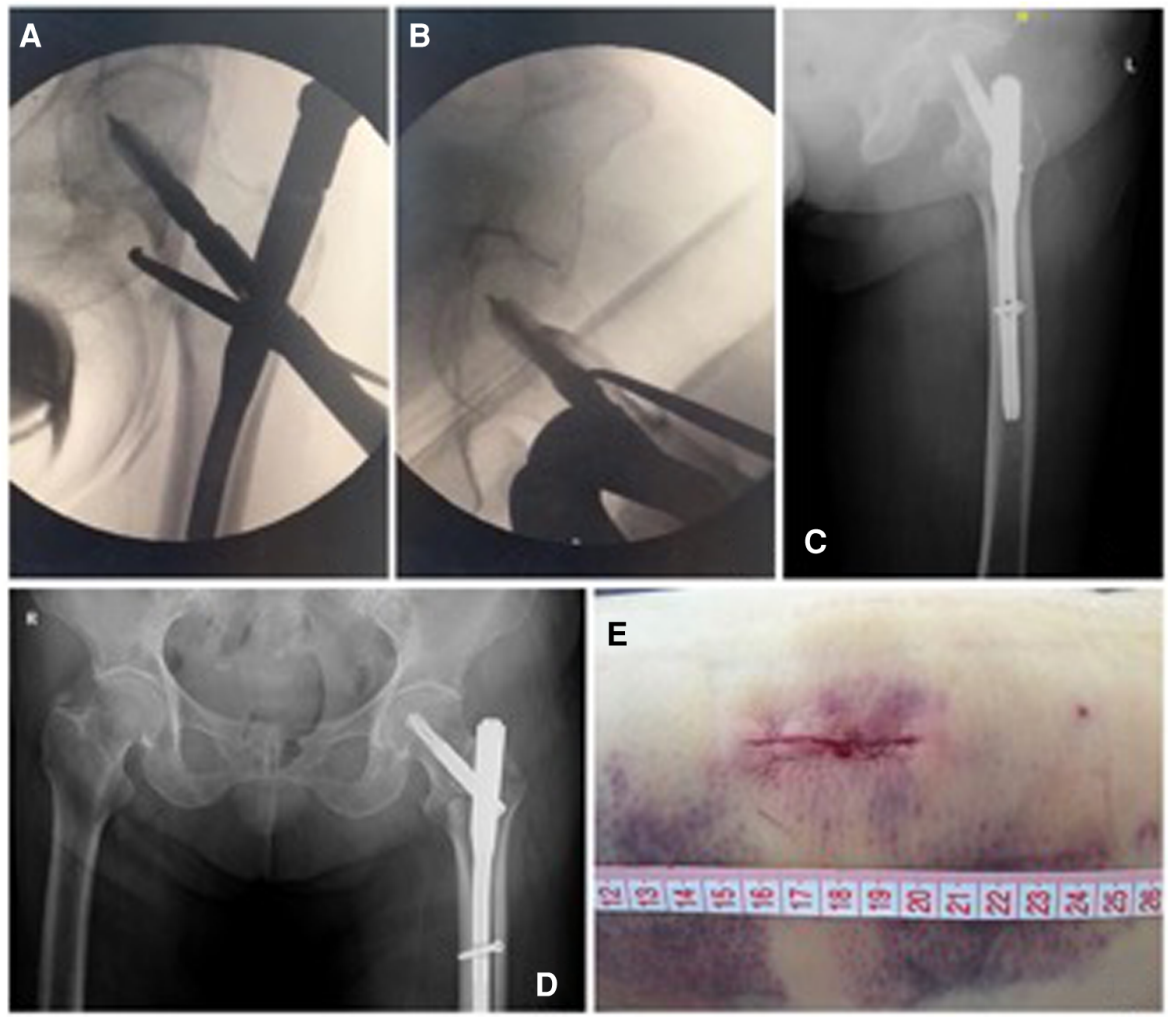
Routine maneuvers during nail fixation. (A,B) Anteroposterior and lateral views of the anatomical reduction and satisfactory fixation by PFNA. (C,D) Postoperative radiographs showing perfect fixation of the intertrochanteric fracture. (E) A minimal incision was used for reduction of the medial arch fragments and helical blade insertion.
